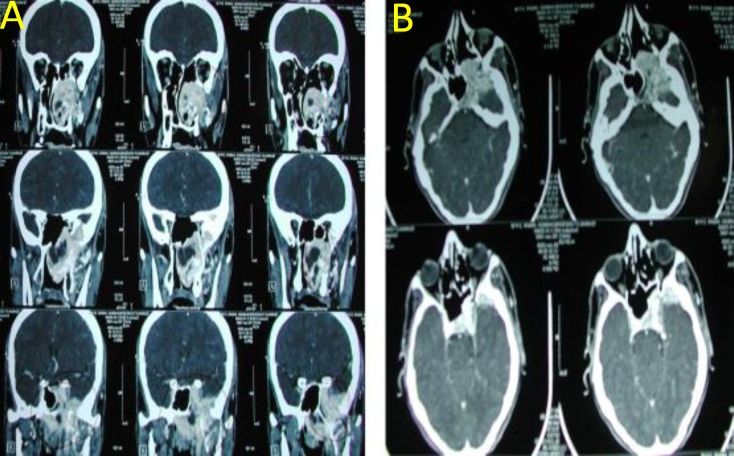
Preoperative coronal (A) and axial (B) views of contrast CT scan of a patient with advanced angiofibroma who was referred for surgery due to nasal obstruction and epistaxis
